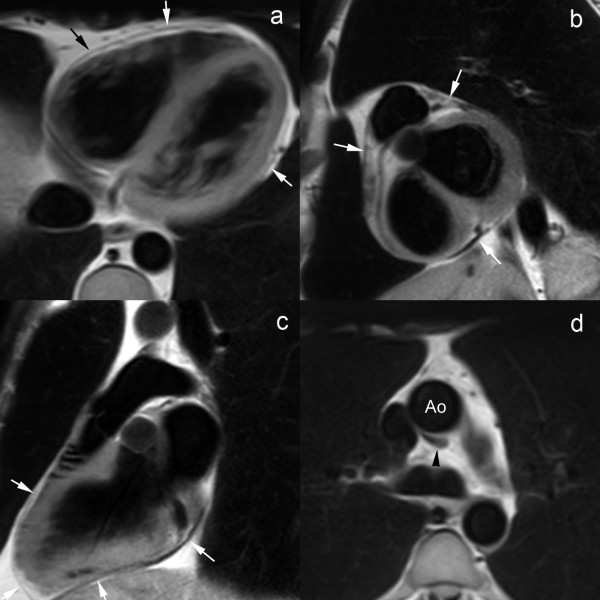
Normal pericardium on T1-weighted fast spin-echo CMR in axial direction. (a), short-axis (b), and vertical long-axis (c), and axial view of superior aortic pericardial recess (d). The normal pericardium is visible as a thin curvilinear structure (arrows) with low signal intensity surrounded by the bright epicardial and mediastinal fat (a-c). Pericardial visualization is often hampered along the free wall of the left ventricle (b). The superior aortic recess, part of the transverse sinus is visible posterior to the ascending aorta, visible as a hypo-intense crescent structure (black arrowhead) (d).
